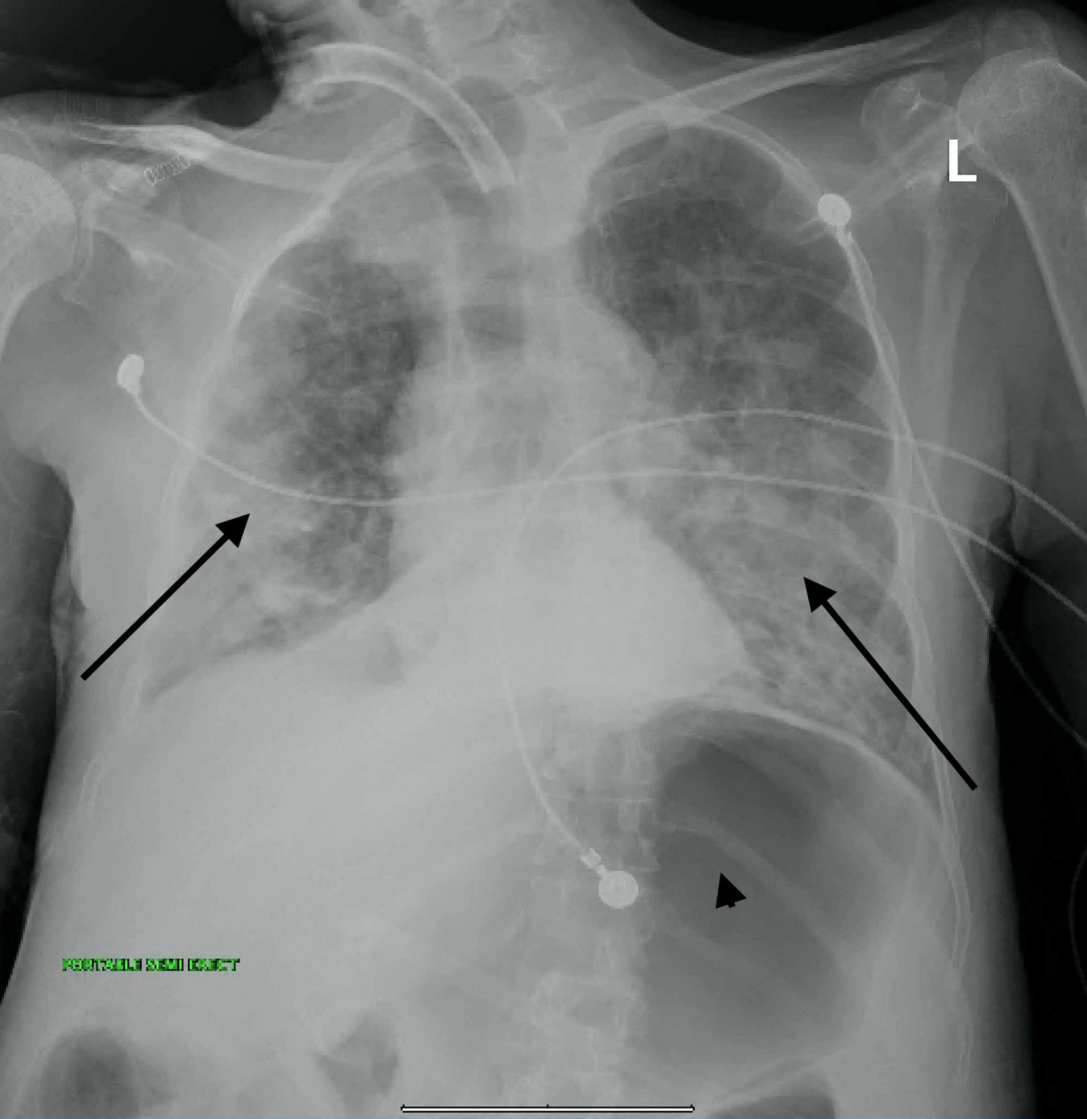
Chest radiograph Arrows point to the areas of extensive bilateral pulmonary infiltrates. Prominent gastric distention with air in the stomach is visible (arrowhead)
